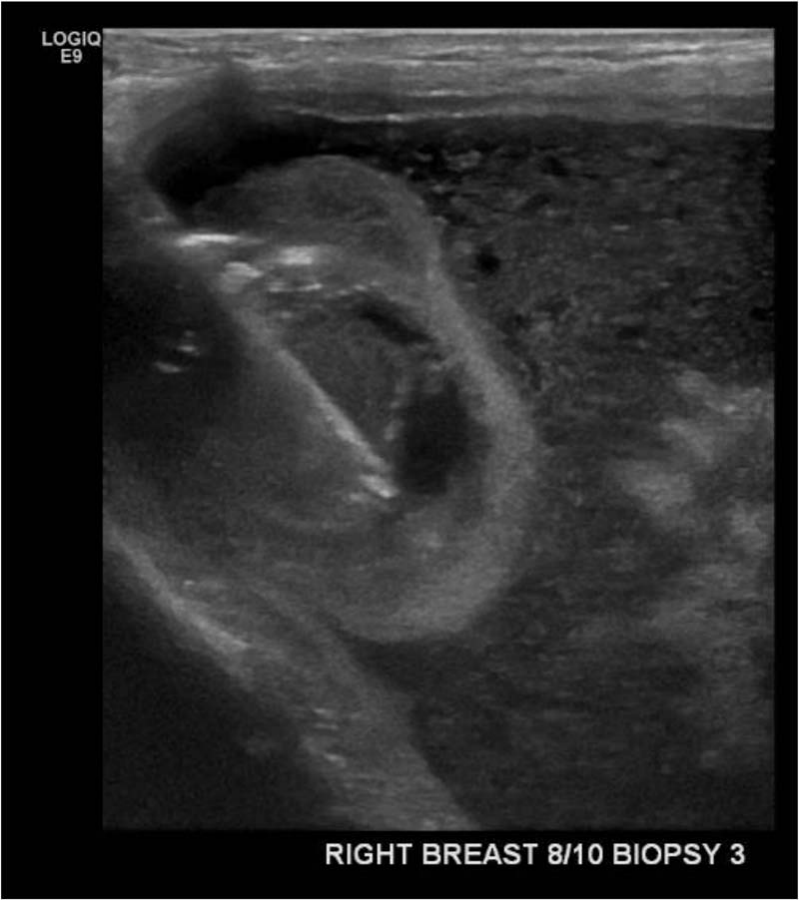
Ultrasound-guided core biopsy of the right intracapsular breast lesion.
